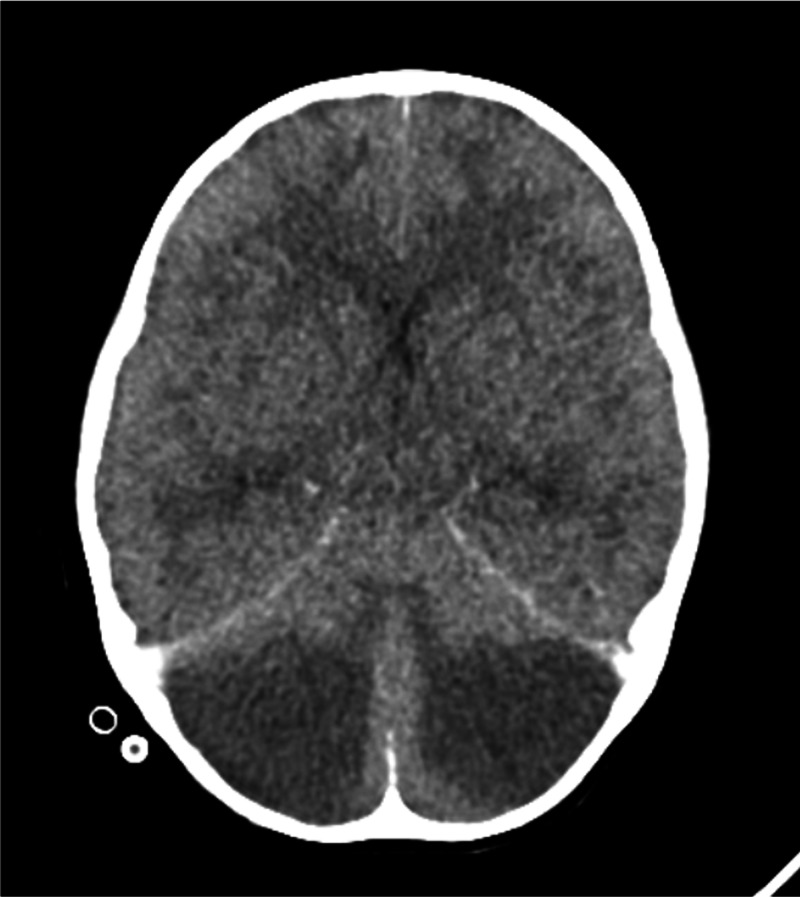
Brain computerized tomography (CT) showed edema in bilateral infratentorial region in both hemispheres.
